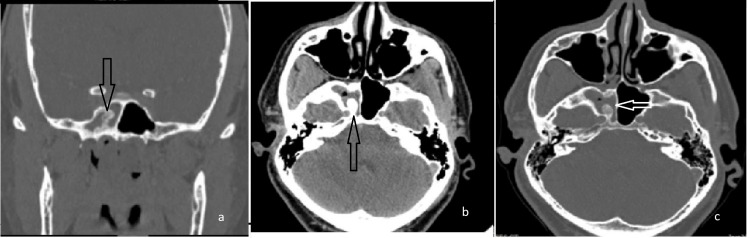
CT scan images of isolated sphenoid inverted papilloma in a young patient (a) coronal view, (b,c) axial views.
